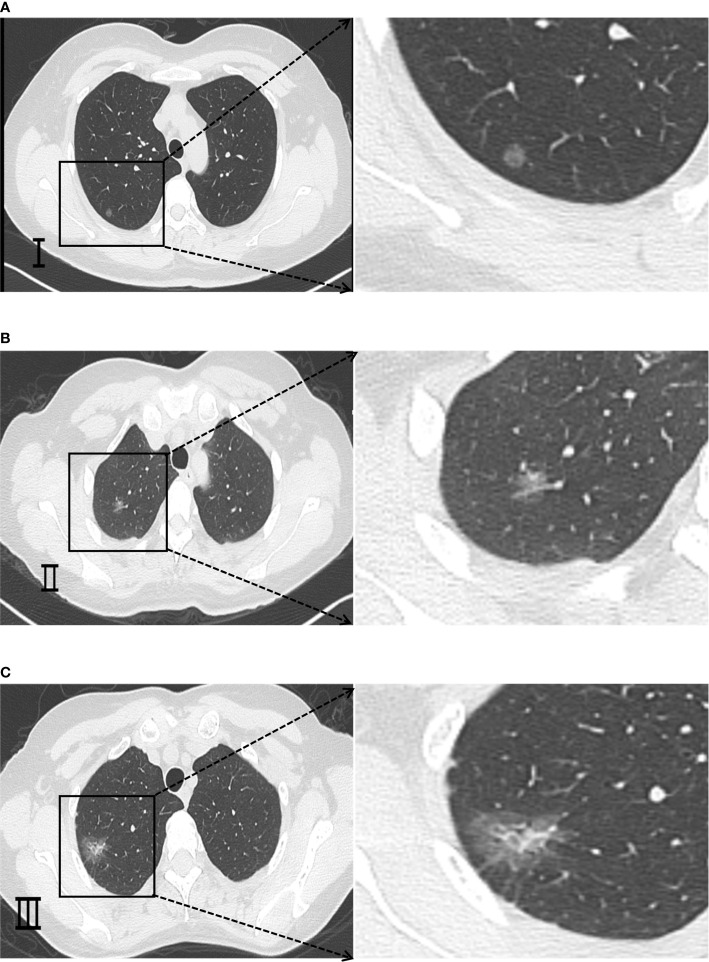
Representative CT imaging findings of GGNs classified according to different morphologies of passing vessels. (A) Type I is a GGN without vascular penetration or with only vessels passing by the side. (B) Type II is a GGN with normal pattern of vessel penetration and no obvious morphological changes in the expected path or thickness of the penetrating vessels. (C) Type III is a GGN with abnormal pattern of vessel penetration, such as thickened, stiffened or twisted vessels and other more complex patterns such as vascular clustering sign, etc.
